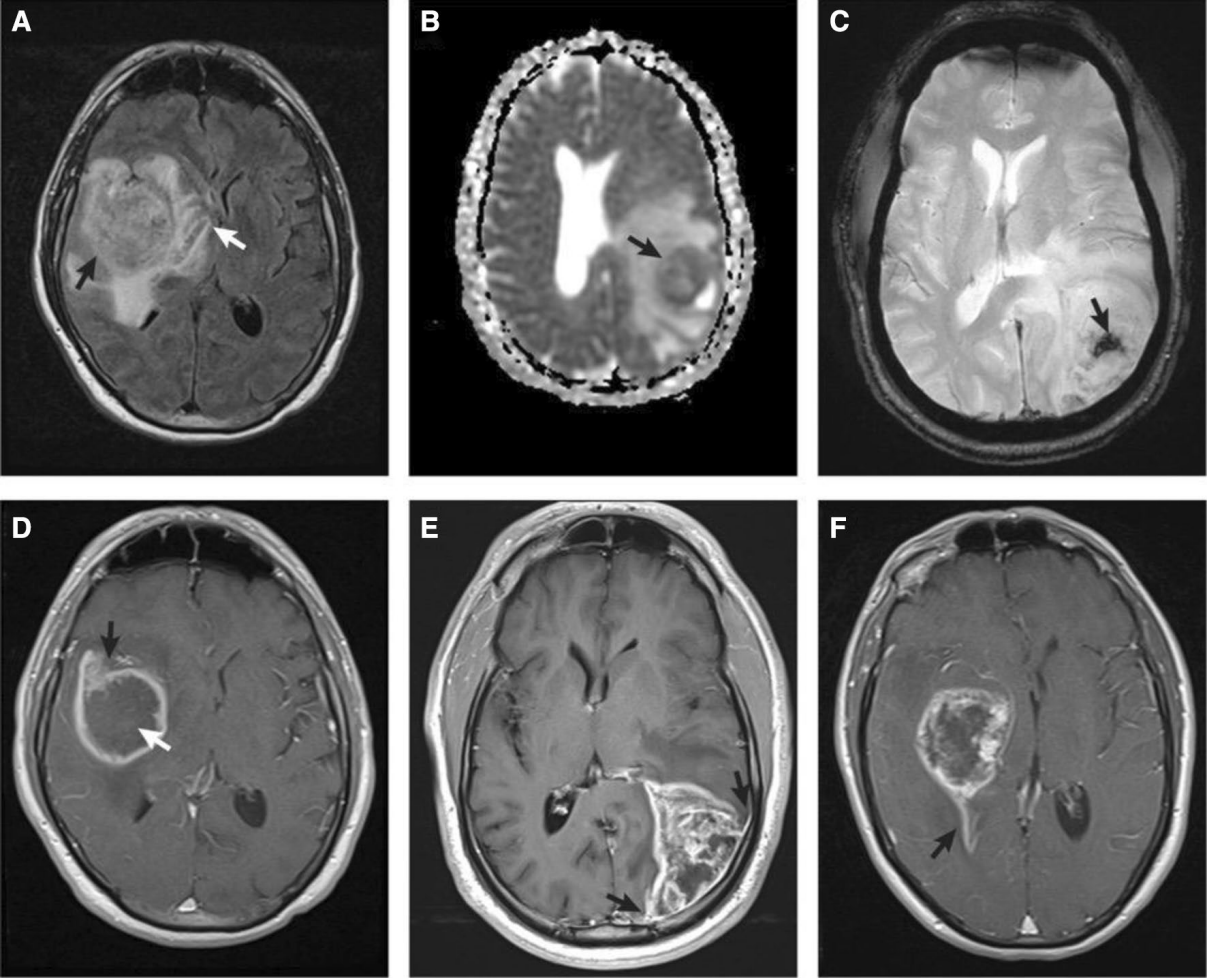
Typical MRI features of gliosarcomas. a FLAIR image demonstrating a centrally isointense mass (black arrow) with extensive surrounding edema extending into the basal ganglia (white arrow). b ADC image showing low diffusion values in the central enhancing portion of the mass (black arrow). c GRE image demonstrating central areas of susceptibility compatible with internal hemorrhage. d–f Postcontrast T1-weighted images showing a thick rim of enhancement (d black arrow) with central necrosis (d white arrow), pial invasion (e black arrows), and ependymal invasion (f black arrow)
